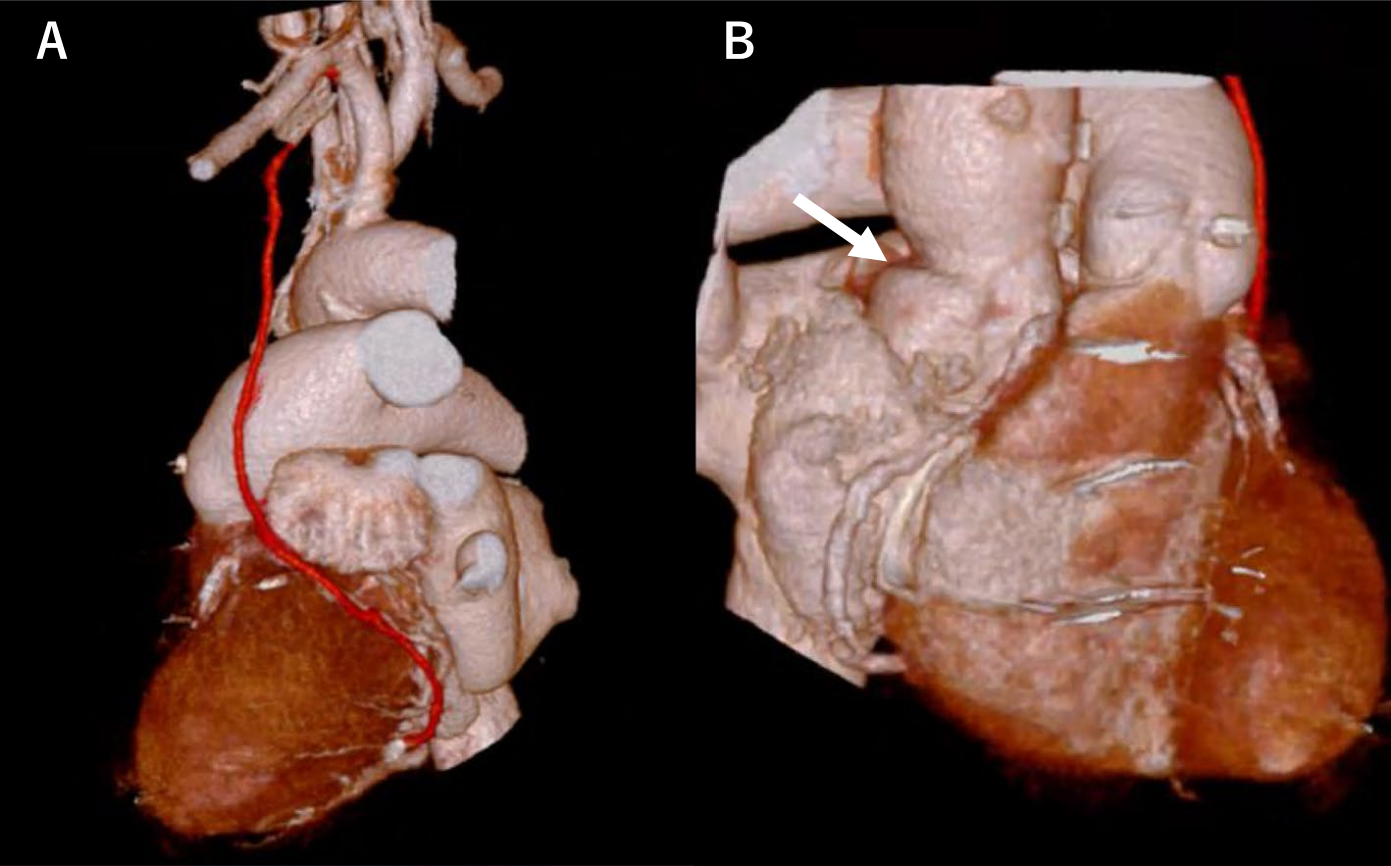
Postoperative computed tomography revealed (A) graft patency and (B) patch enlargement (white arrow)
